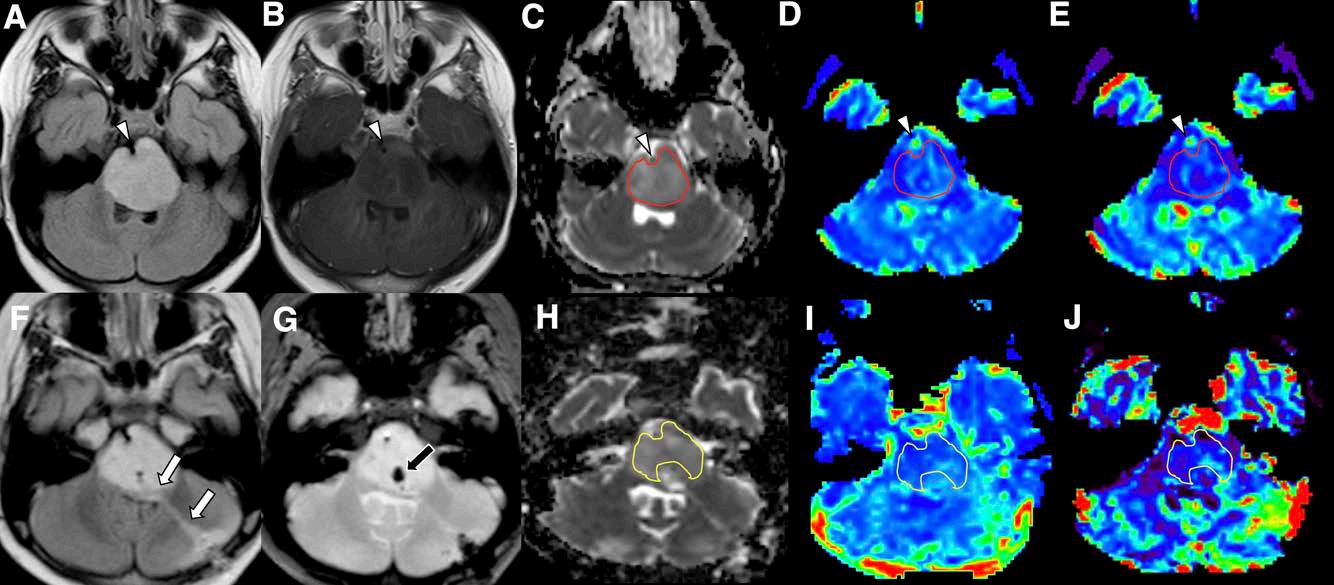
Example of region-of-interest (ROI) placement (a–e: prebiopsy MRI, f–j: postbiopsy MRI) On the prebiopsy MRI, the expansile pontine diffuse midline glioma, H3K27-altered appears hyperintense on the FLAIR image (a) with scarce enhancement on the postcontrast T1-weighted image (b). ROIs are placed on the ADC map (c), corrected rCBV map (d), and rCBF map (e) while avoiding the basilar artery (arrowheads). the biopsy tract (f, white arrows) and hemorrhagic focus (g, black arrow) are observed on the postbiopsy FLAIR MRI image (f) and T2*-weighted image (g). ROIs are placed on the ADC map (h), corrected rCBV map (i), and rCBF map (j) while avoiding basilar artery and the postbiopsy changes. DSC = dynamic susceptibility contrast, ADC = apparent diffusion coefficient, FLAIR = fluid-attenuated inversion recovery, rCBV = relative cerebral blood volume, rCBF = relative cerebral blood flow.
